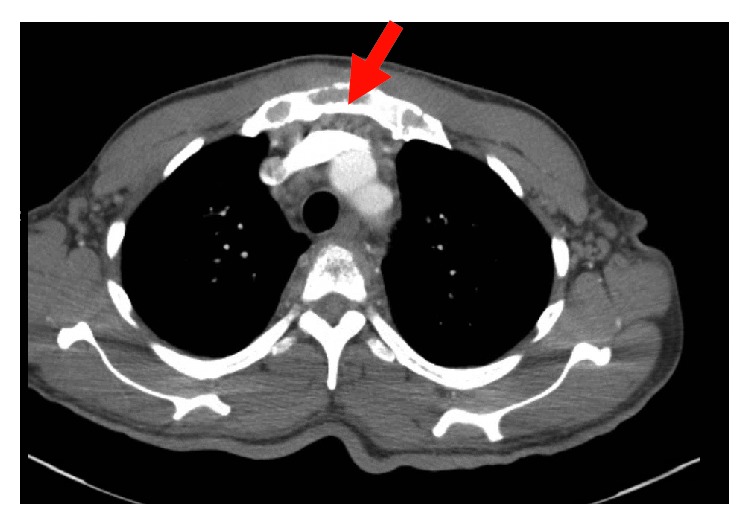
CT chest with contrast showing lesions in the ribs and sternum.
